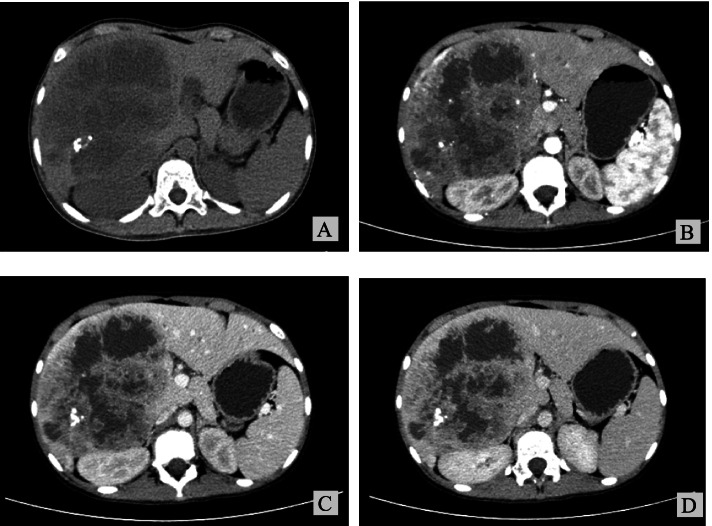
Preoperative CT. 1A Plain CT scan shows a large mass in the right lobe of the liver. 1B No internal enhancement was observed in the arteriolar mass on enhanced CT, with multiple cystic changes, faint drifting signs, and obvious enhancement of the surrounding liver parenchyma. 1C Portal vein phase on enhanced CT. 1D Delay period of enhanced CT
